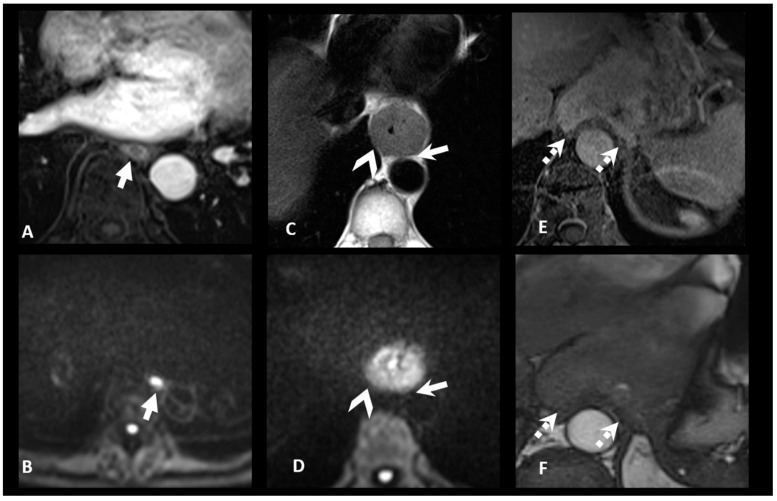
Contribution of MRI for T staging. The transverse MRI images in T1WI FS GD (A) and DWI (B) show a small tumor in the middle third of the esophagus without invasion of the adjacent fat, classified as MR-T1/2. The T2WI and DWI transverse MRI images (C,D) show a tumor in contact with right pleura (arrowhead) and focally with aorta (arrow), without invasion, classifying this tumor as MR-T3. The transverse MRI images in T1WI FS GD show a large tumor of gastroesophageal junction Siewert 3 (E,F) invading diaphragmatic pillars (dashed arrow) with loss of peripheric hyposignal on kinetic SSFP sequences, classifying the tumor as MR-T4a. Images acquired on a 3 T-scanner (Magnetom PrismaFit, Siemens Healthcare). WI: weighted imaging; DWI: diffusion-weighted imaging; FS GD: fat sat with gadolinium; and SSFP: steady state free precession.
